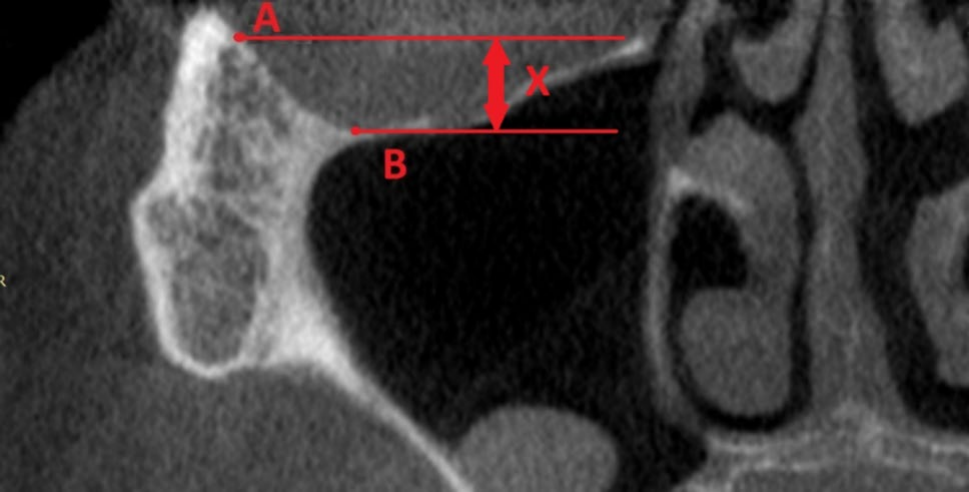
Reference points in the frontal plane on cone-beam computed tomography (CBCT) were the lowest (B) and the most lateral point (A) in the lower wall of the orbit
